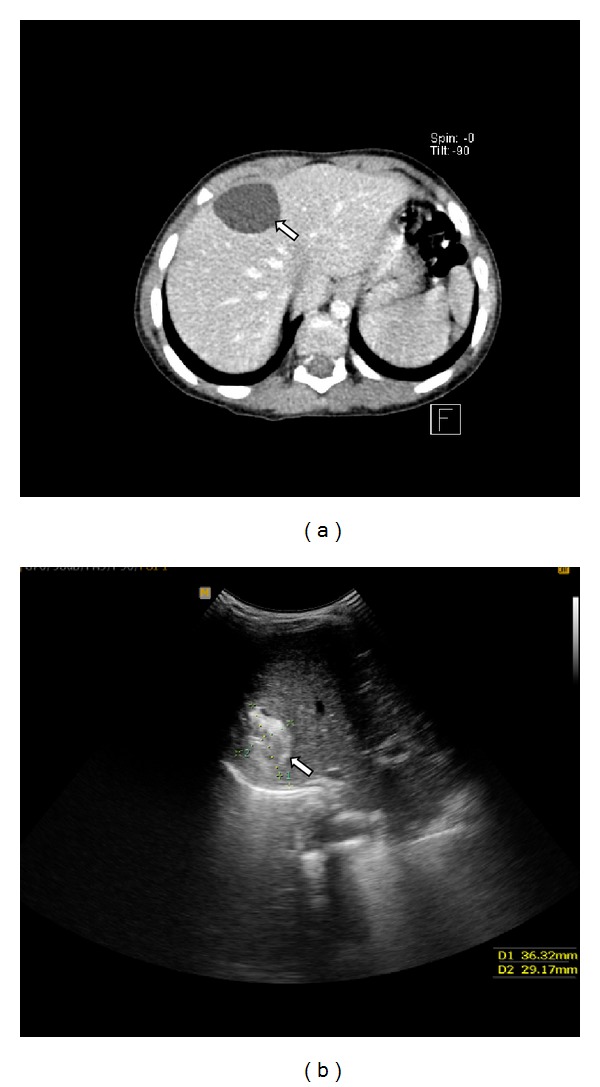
(a) CT shows a simple 3.7 × 2.8 cm subcapsular liver cyst in median segment of left lobe (left arrow). (b) US shows a 3.6 × 3.2 cm heterogeneous lesion in right lobe of liver (left arrow).
